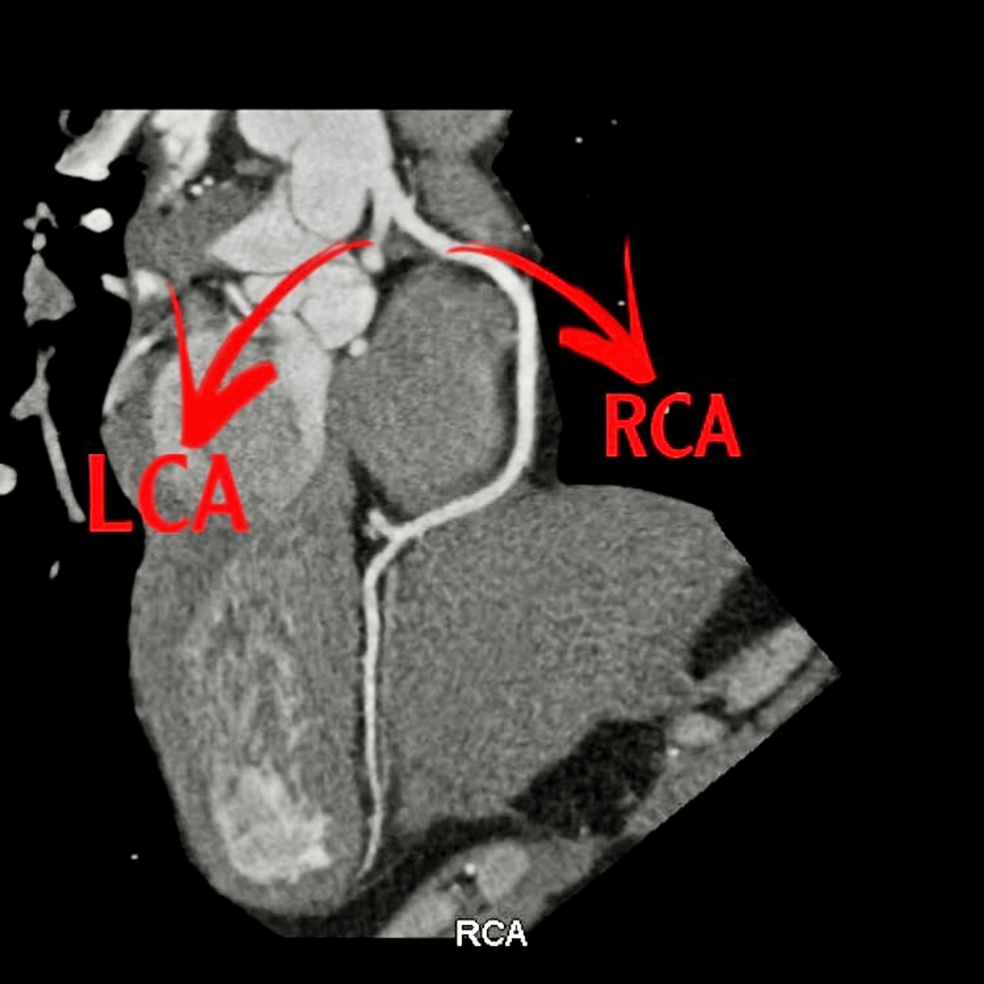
A cardiac computed tomography scan shows bilateral perivascular ground-glass opacities, indicating infection, along with an anomalous left main coronary artery (LCA) originating from the right coronary artery (RCA) with a retro-aortic course
